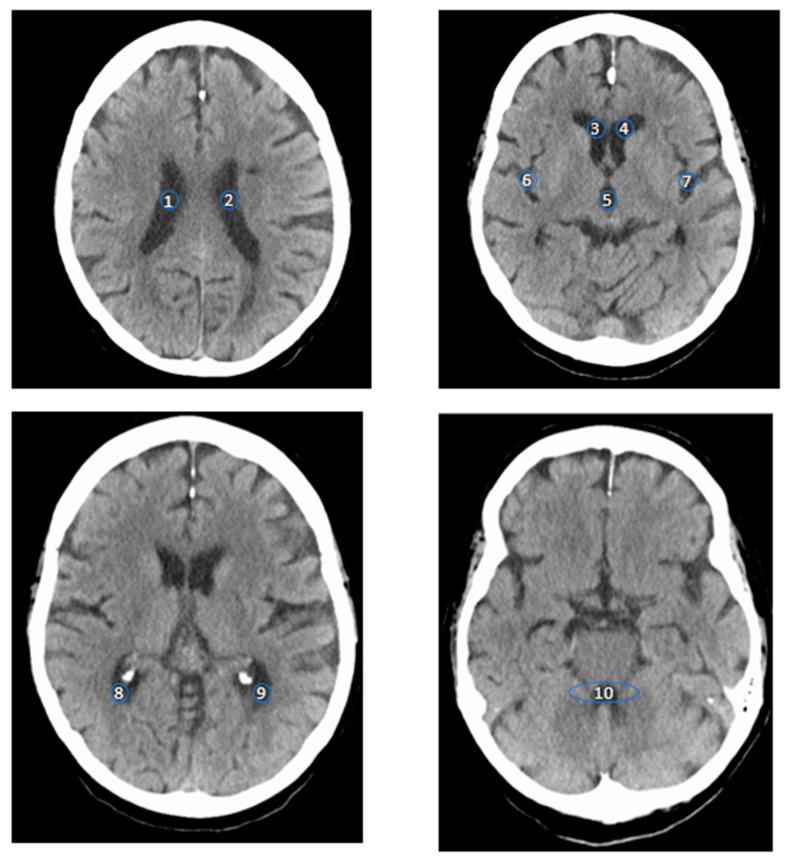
The four images show axial CT scans of the brain at different axial levels. The numbers indicate where the measurements were conducted, as described below: (1) the central part of the right lateral ventricle; (2) central part of the left lateral ventricle; (3) anterior horn of the right lateral ventricle; (4) anterior horn of the left lateral ventricle; (5) third ventricle (III); (6) right sylvian fissure; (7) left sylvian fissure; (8) posterior horn of the right lateral ventricle; (9) posterior horn of the left lateral ventricle; and (10) fourth ventricle (IV).
